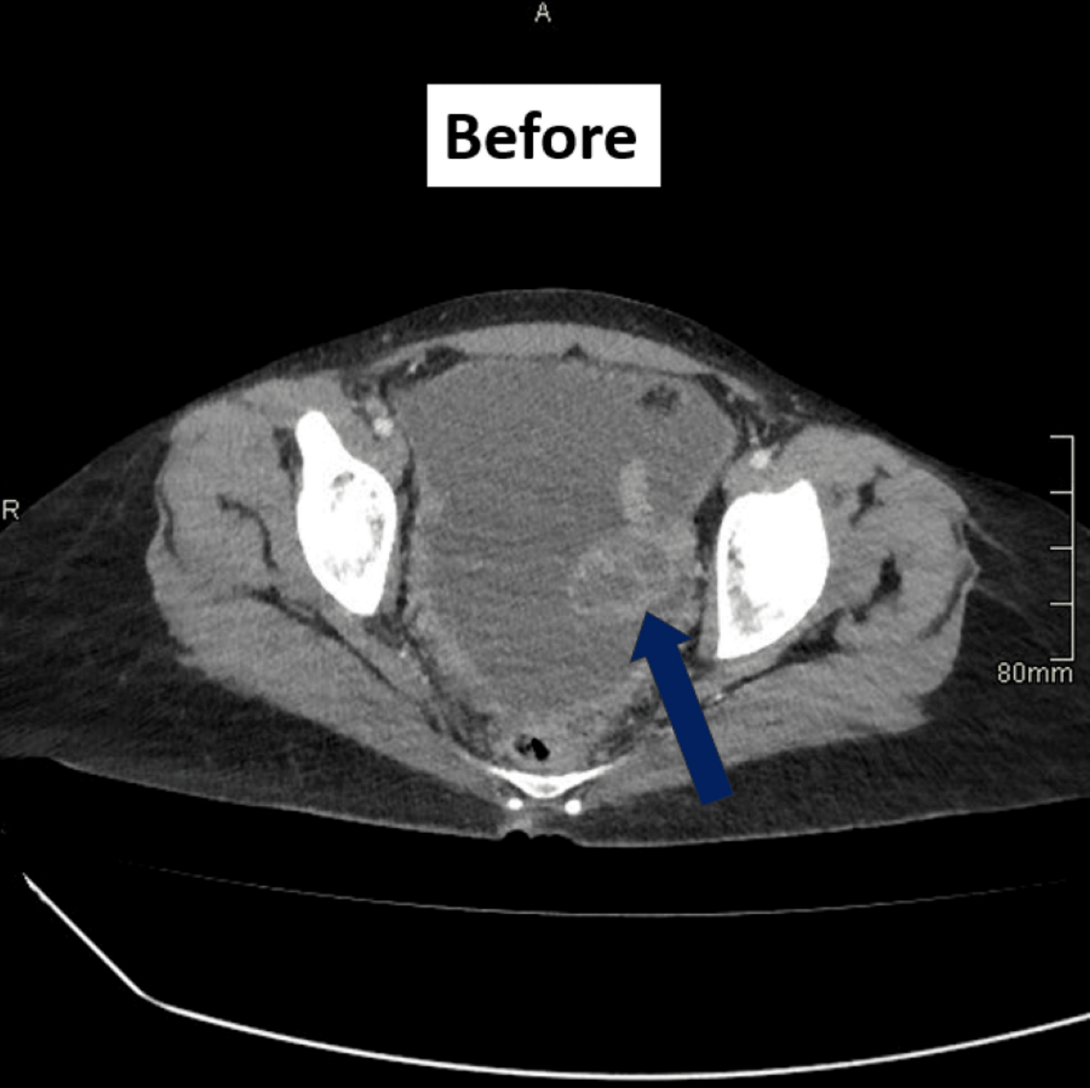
CT scan before treatment. The arrow points to a complex, loculated cystic mass in the left adnexa, present at initial presentation.
